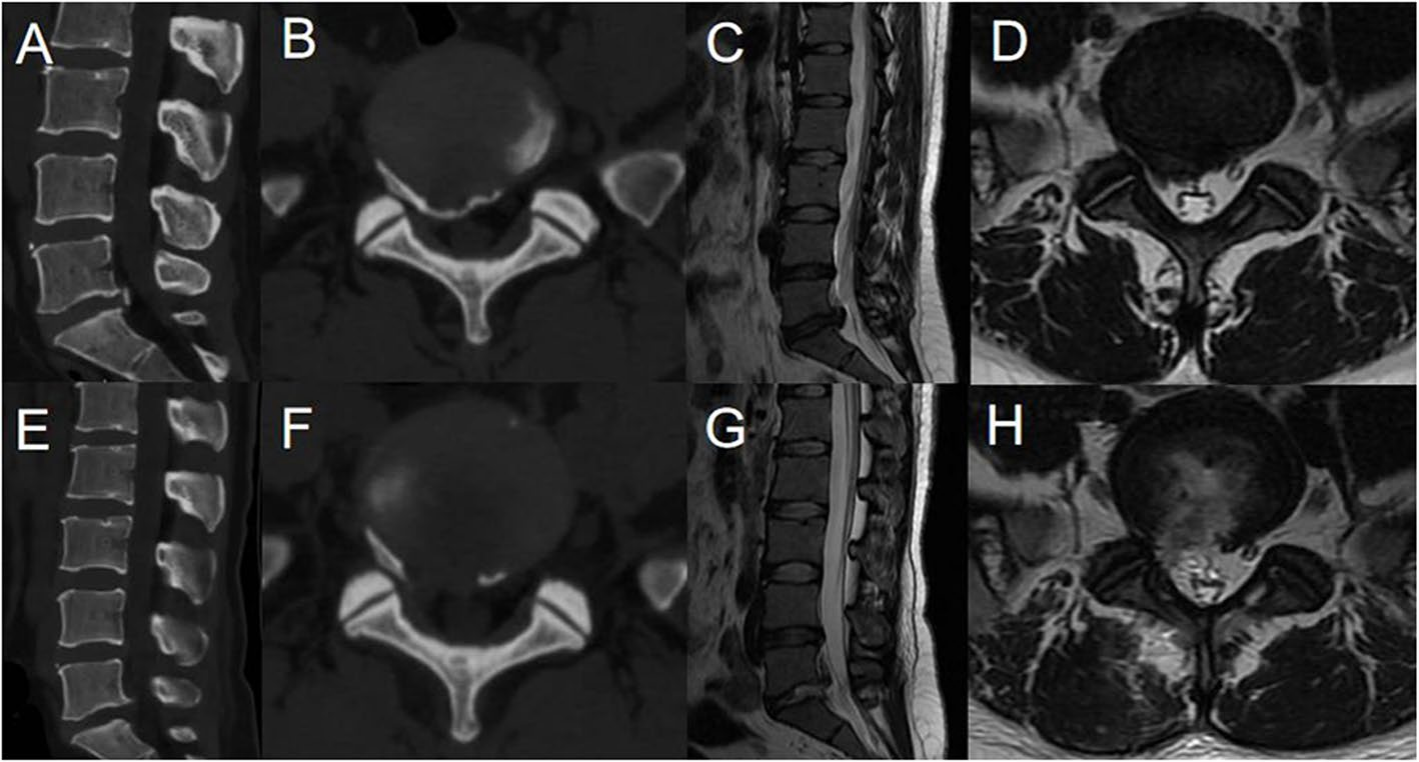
Pre- and post-operative MRI and CT (A-H). A-D Preoperative CT and MRI revealed lumbar disc herniation combined with calcification. E–H Postoperative CT and MRI showed that the calcified intervertebral disc was removed and the compressed nerve root had been relieved by PEID
